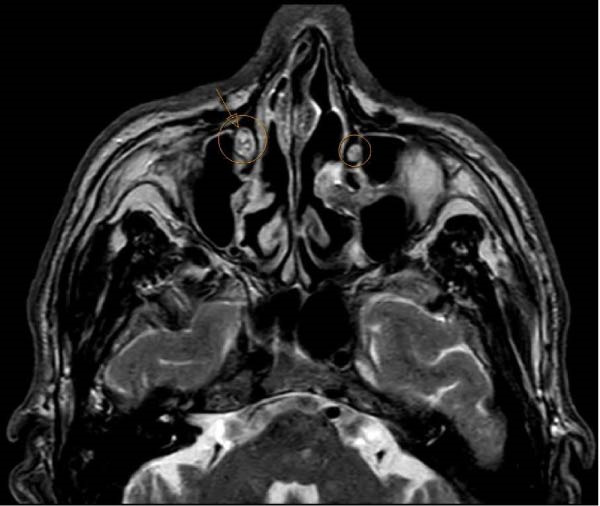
Preoperative MRI, lacrimal sac invasion.
